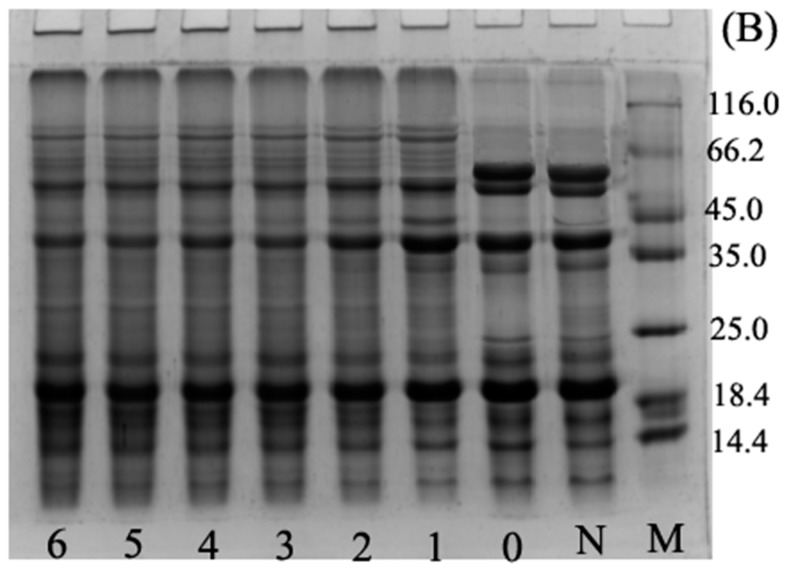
SDS-PAGE profiles of BBPI by wet heating (A) and ultrasound treatment Maillard reaction (B). Lane M: maker, lane N: native BBPI, lane 0: BBPI of ultrasound treatment, lanes 1–6: wet Maillard reaction (A) and ultrasound treatment Maillard reaction (B) for 1–6 h, respectively. The concentration of protein solution in each lane of the gels was 5 mg/mL.
